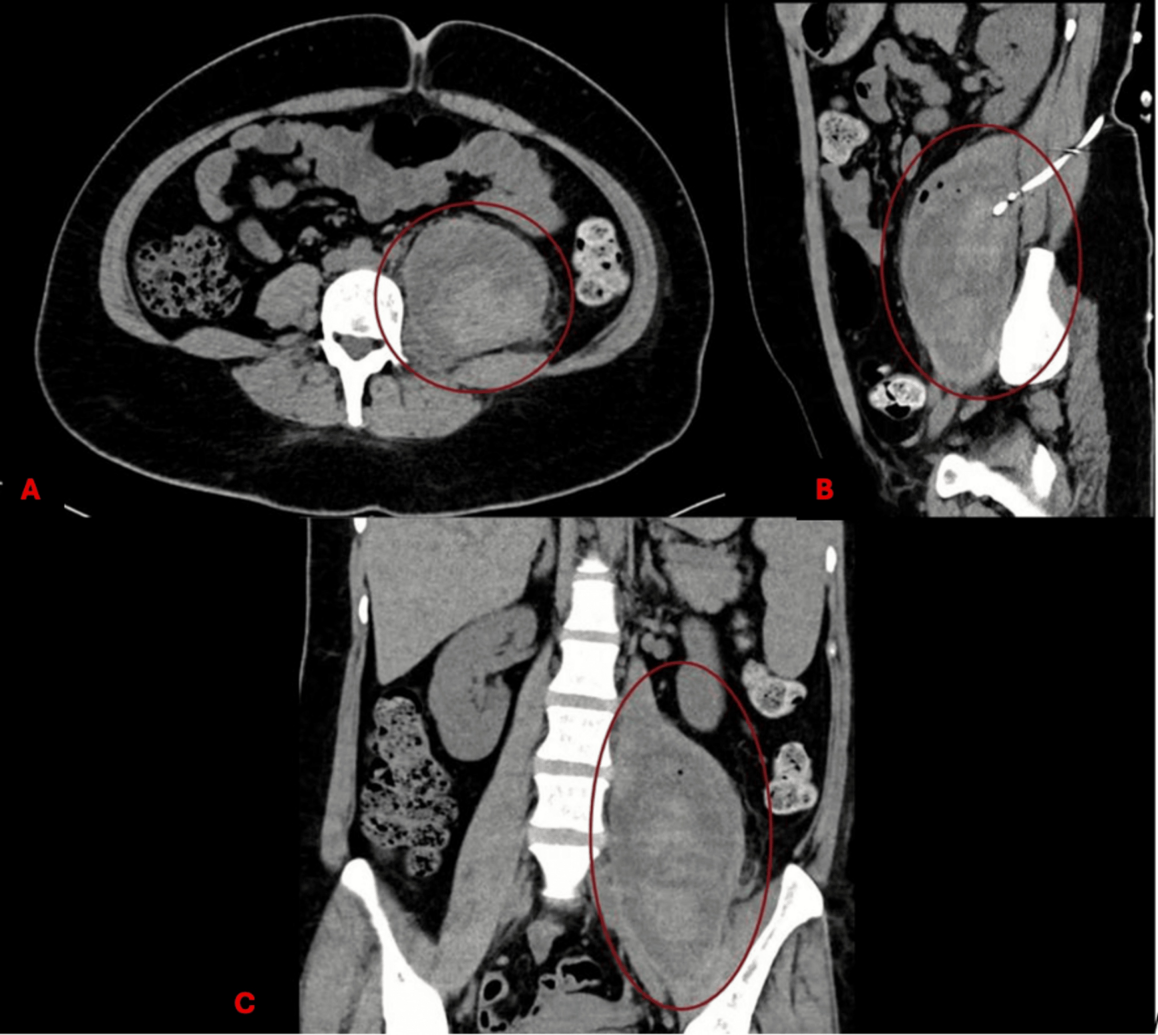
Hematoma in the iliopsoas vizualized via a contrast-enhanced computed tomography (CT) scan in the bone window at the one-month follow up. The CT scan shows a decrease in the initial volume to 352 cc. Cross-section (A) and sagittal section (B) show the correct placement of drainage in the hematoma. Pane C showcases the coronal section. Cc: cubic centimeters
